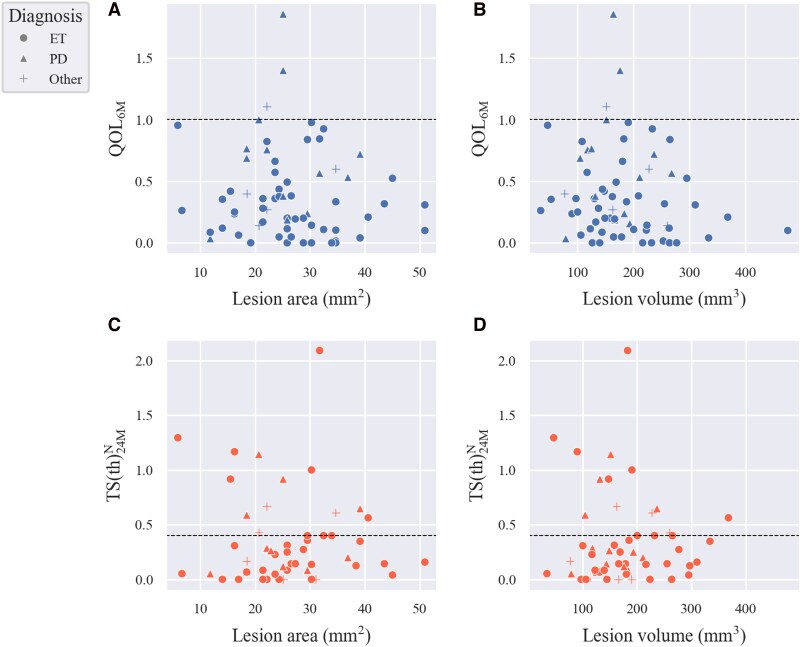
Lesion area and volume evaluation. QoL evaluation at 6 months FU (*N* = 66) with respect to lesions (A) area at ACPC plane and (B) total volume, at POD1 MRI. Tremor evaluation at 24 months FU (*N* = 54) with respect to lesions (C) area at ACPC plane and (D) total volume, at POD1 MRI. Success-failure threshold as applied in the success criteria (dashed black line). Each point represents a patient (*N* = patients). FU, follow-up, TS(th)^N^, tremor score of treated hand normalized to baseline, COM, centre of mass; ET, essential tremor; PD, Parkinson’s disease; POD1, postoperative day 1.
